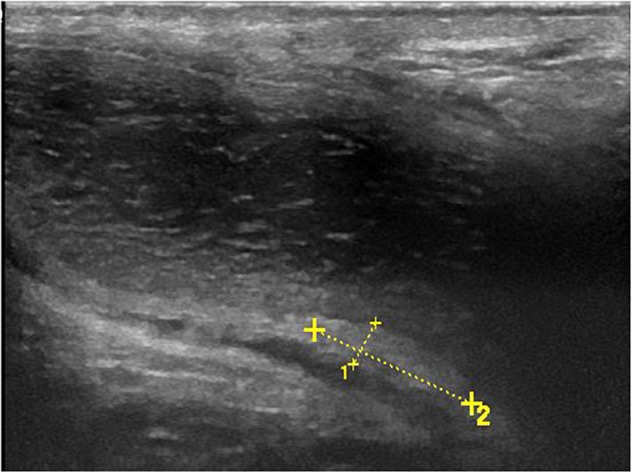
Longitudinal sonogram (ventral access) of a palpable dorsal nodule. This nodule was not detected by the sonography in the flaccid penis. The sonography in erect penis shows obvious dorsal tunica albuginea thickening at the root of penis.
